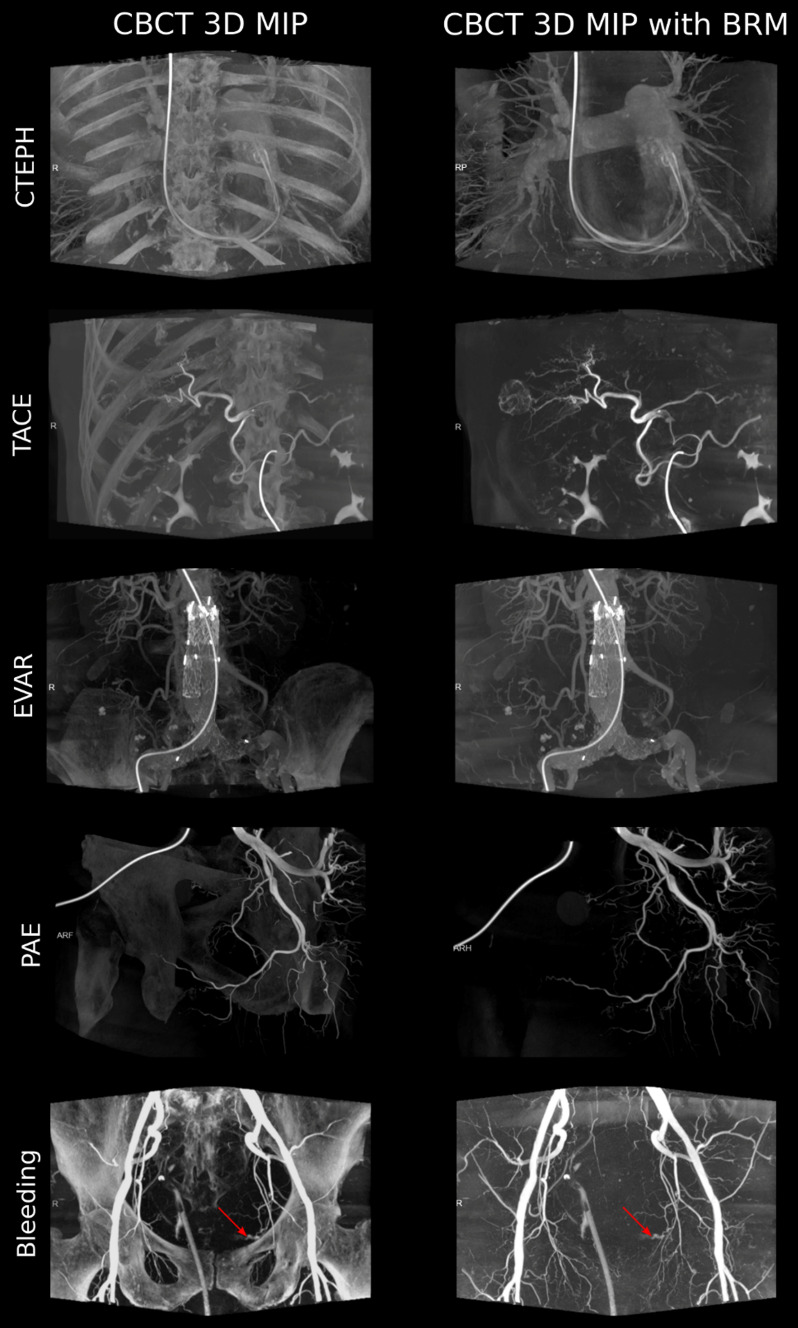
The study compares 3D maximum intensity projections (MIP) of cone beam CT (CBCT) scans, both with and without bone removal (BRM). These scans were performed for various medical procedures. One scan of the thorax was conducted to treat chronic thromboembolic pulmonary hypertension (CTEPH). Another scan of the upper abdomen was used for transarterial chemoembolization (TACE). A scan of the lower abdomen was carried out for endovascular aneurysm repair (EVAR). Additionally, scans of the pelvis were performed for prostate artery embolization (PAE) and for the detection of bleeding. The red arrows point to the bleeding
